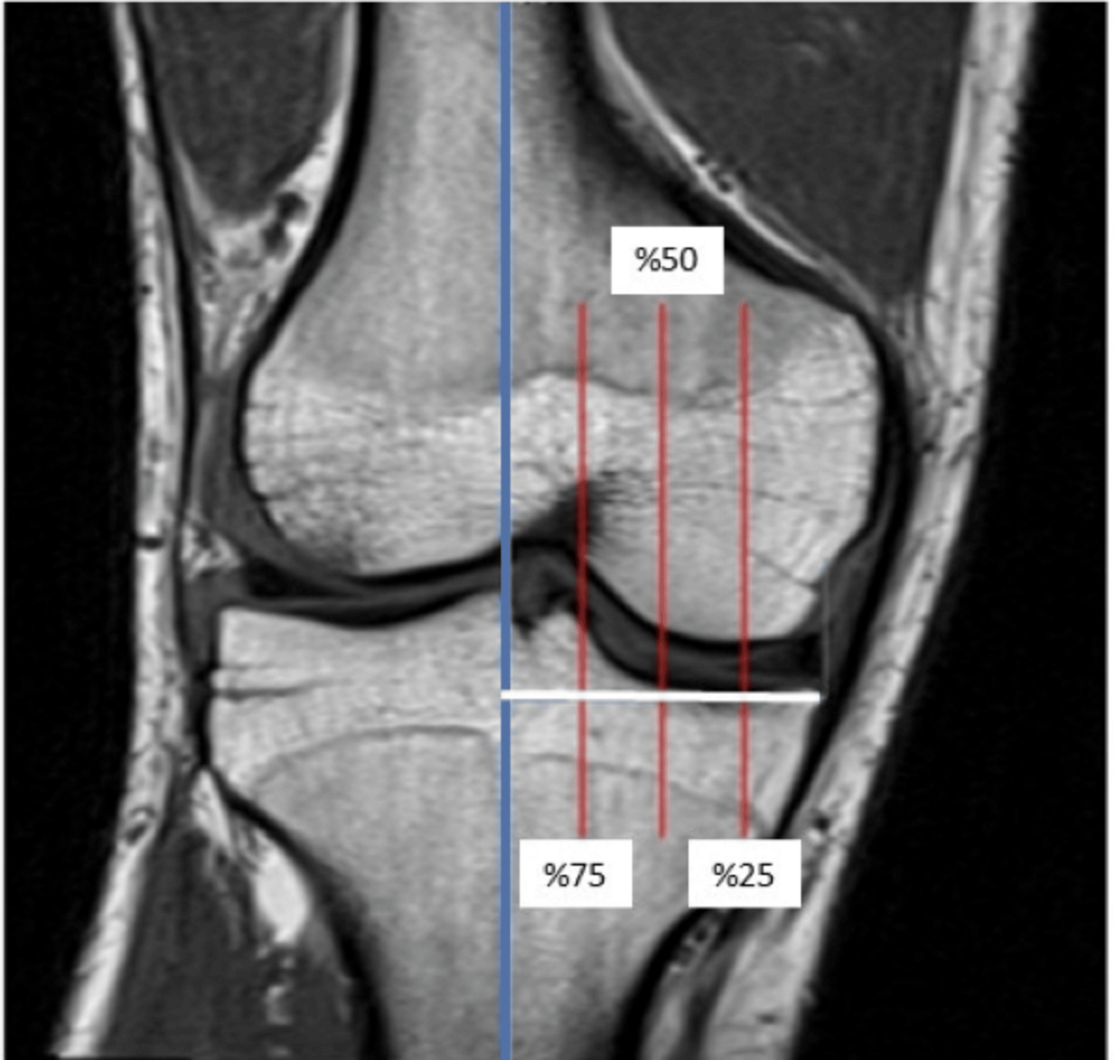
Determination of sections in MRI scans.
